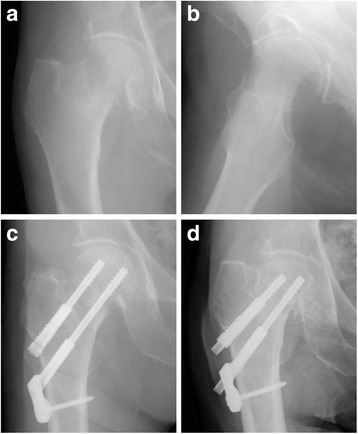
Radiographs showing a case of non-union of femoral neck fracture after internal fixation. Excessive sliding of Dual SC Screws was observed. (a, b preoperative, c postoperative, d ;4 months postoperatively)
